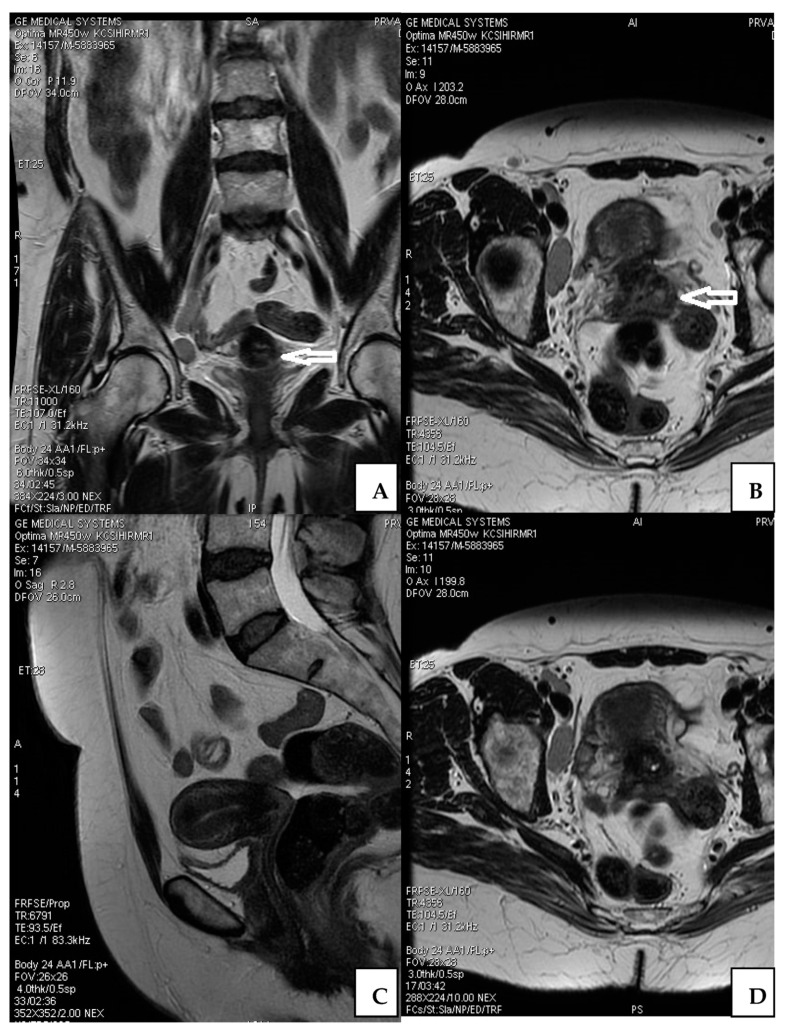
MRI of the abdomen and pelvis after conization. Cervical lesion (arrow) on diferent imaging presentations (A,B); Endometrium without detected tumor lesions (C); Adnexa without tumor lesion (D).
